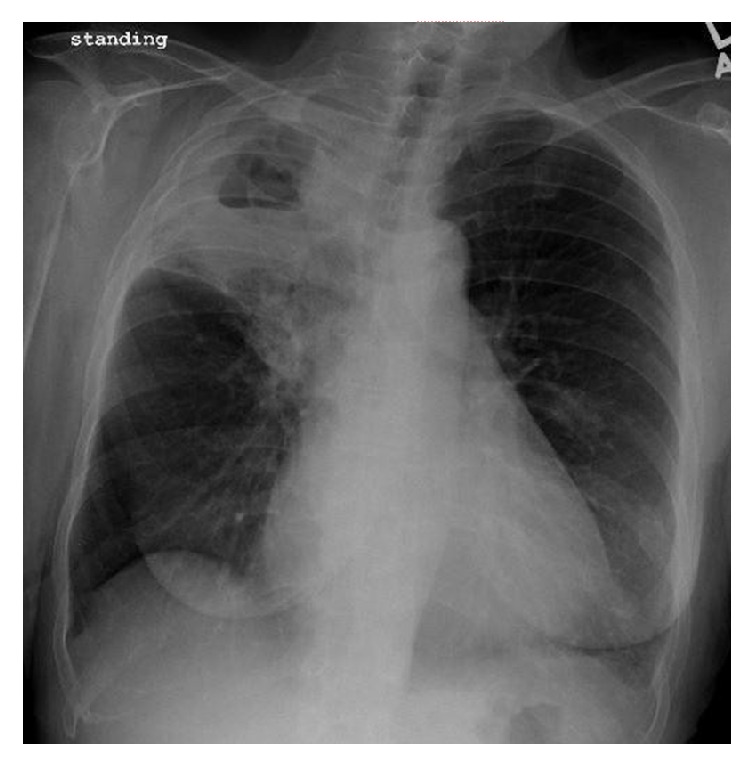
Chest X-ray with right upper lobe cavitating pneumonia.
